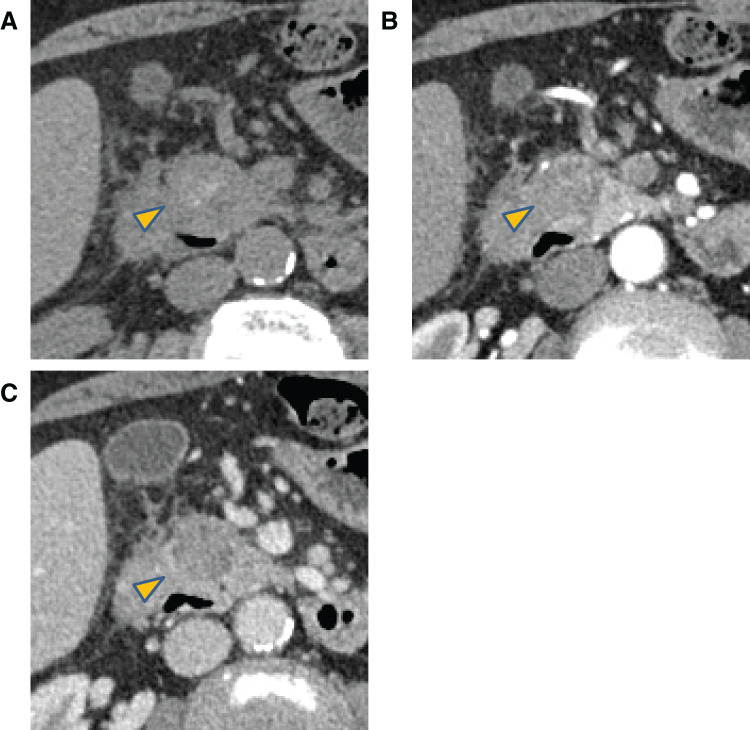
Dynamic CT images. (A) Image obtained during CT without contrast. (B) Arterial phase image. (C) Portal phase image. A 24-mm × 19-mm mass (arrowhead) with high attenuation areas and poor internal contrast enhancement is observed in the groove area. Contrast enhancement is observed during the delayed phase in the peripheral region of the mass. Additionally, increased fat density is observed around the descending part of the duodenum adjacent to the mass; however, no evidence of contrast agent extravasation is observed.
